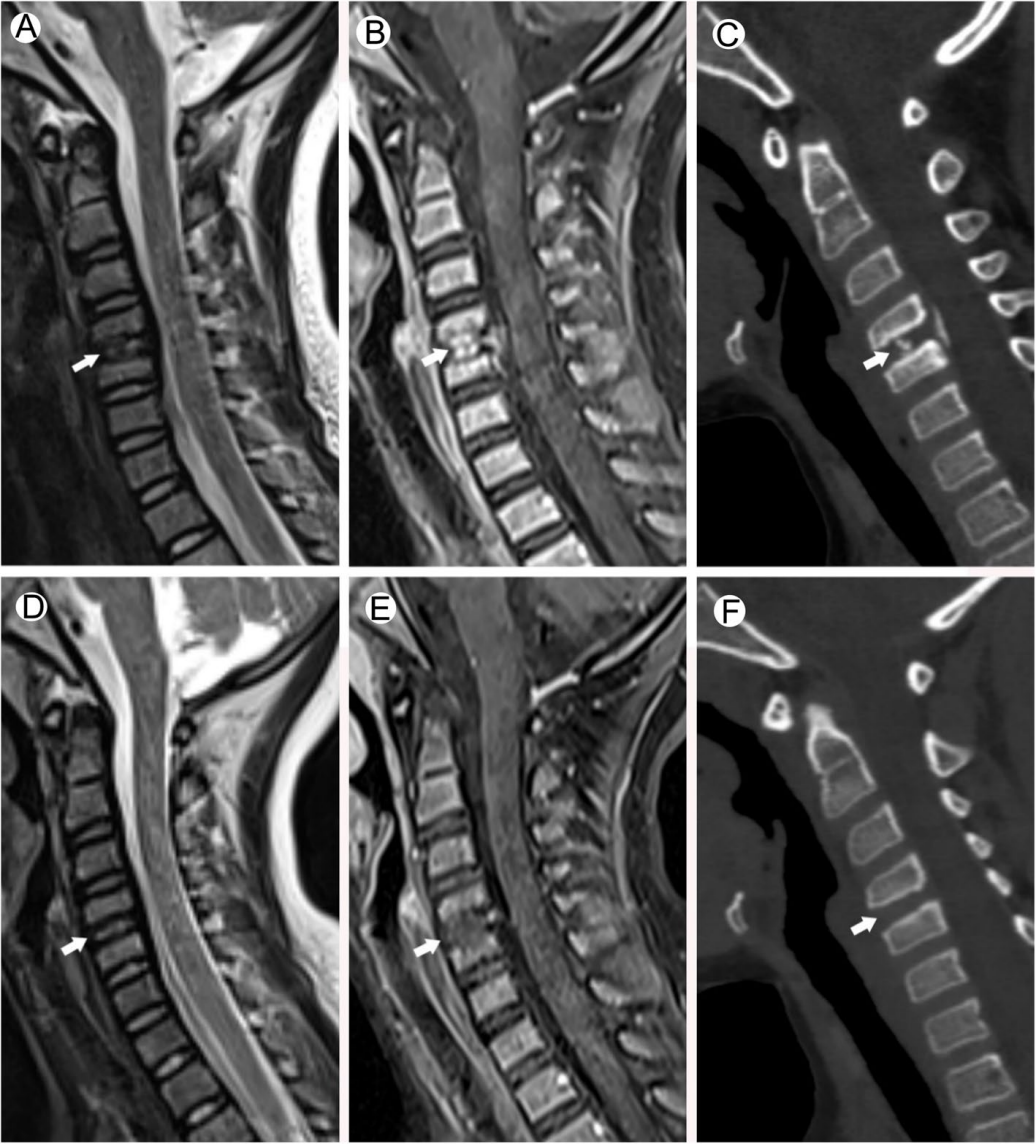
Radiological imaging at admission (A, B, C). The sagittal T2WI (A) of the child at admission shows a partially reduced signal in the intervertebral disc. The sagittal T1-FS enhanced examination (B) shows patchy enhancement in the nucleus pulposus from the C4/C5 intervertebral disc and the corresponding posterior longitudinal ligament area. Cervical CT sagittal view (multi-planar reformation, MPR image) (C) shows calcification of the C4/C5 intervertebral disc, ossification of the posterior longitudinal ligament at the C4/C5 level, shortened longitudinal axes of the vertebral bodies of C4 and C5, and a heterotrophic calcific deposit at the endplate of the opposite edges of the vertebral bodies. After 8 months of follow-up, the sagittal images of the same patient (D, E, F) show that the signal from the C4/C5 disc is restored, the enhancement of the original disc and posterior longitudinal ligament disappears, and the disc calcification and posterior longitudinal ligament ossification also completely disappears
